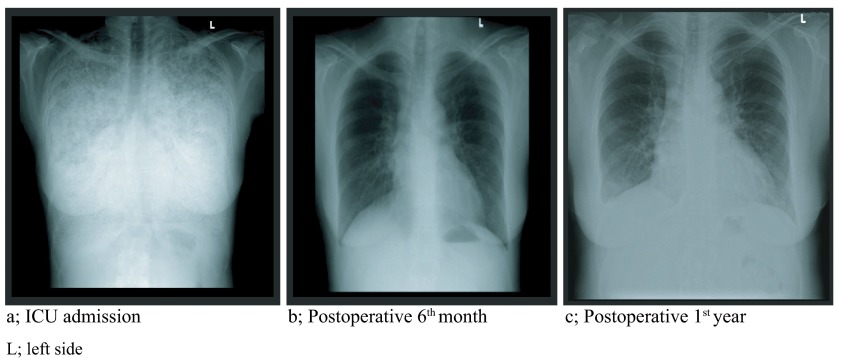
Chest X-rays of the PAM diagnosed patient. a; ICU admission (typical chest image of PAM)
